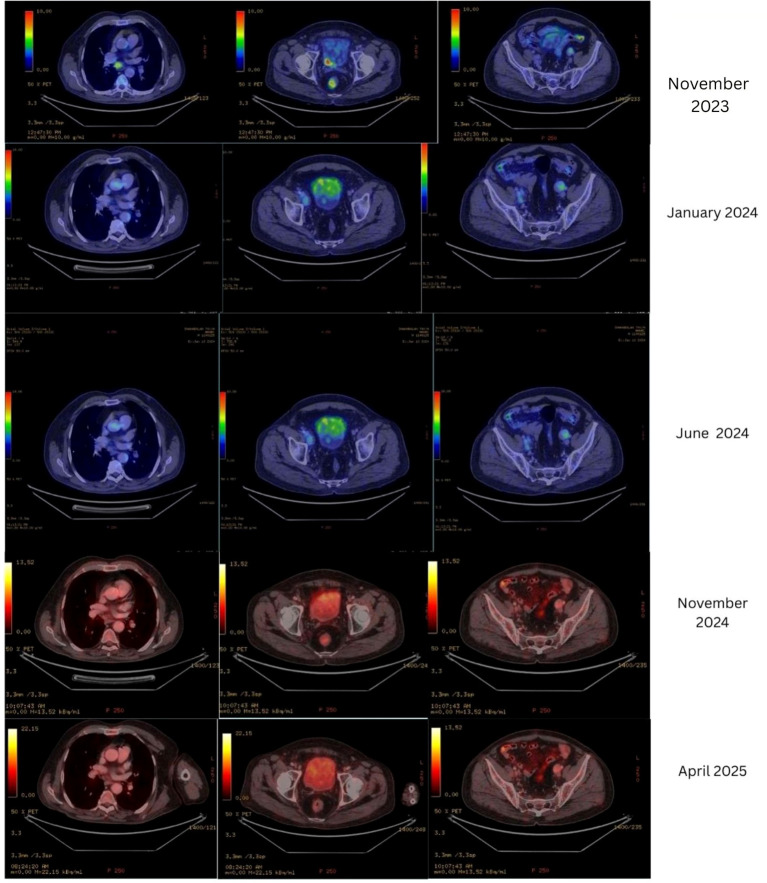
Changes in PET-CT across time points.
